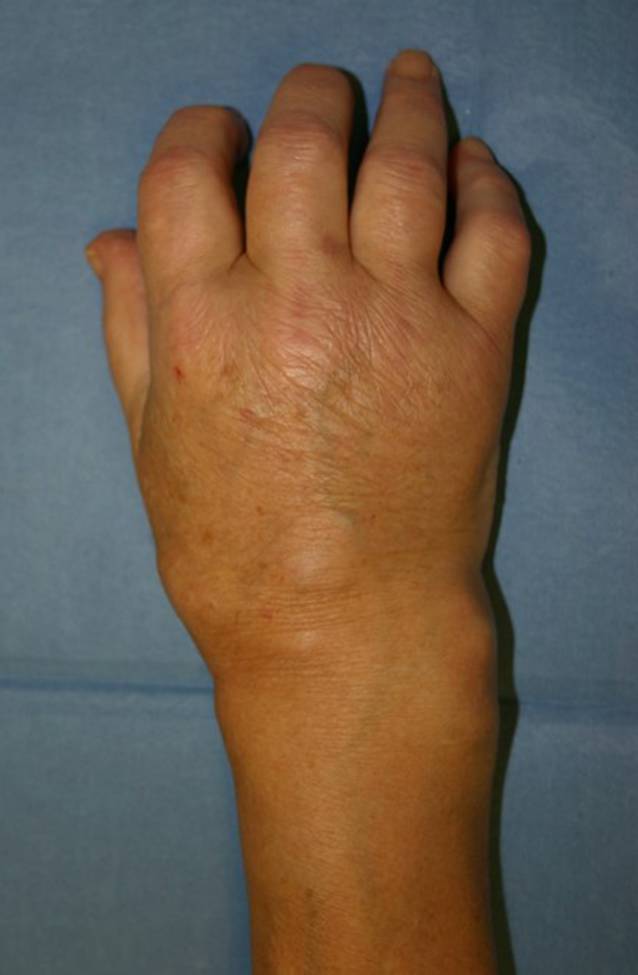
#### Sonographie und Punktat des Ergusses
